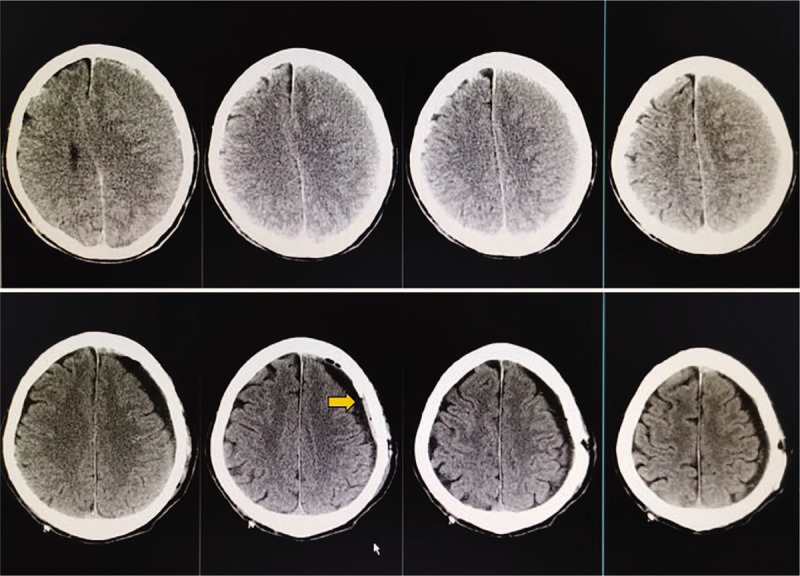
Upper: Preoperative CT. Lower: Postoperative CT. The orange arrow shows the fully expanded subarachnoid space, and the drainage tube inside the hematoma cavity is pushed against the skull.
